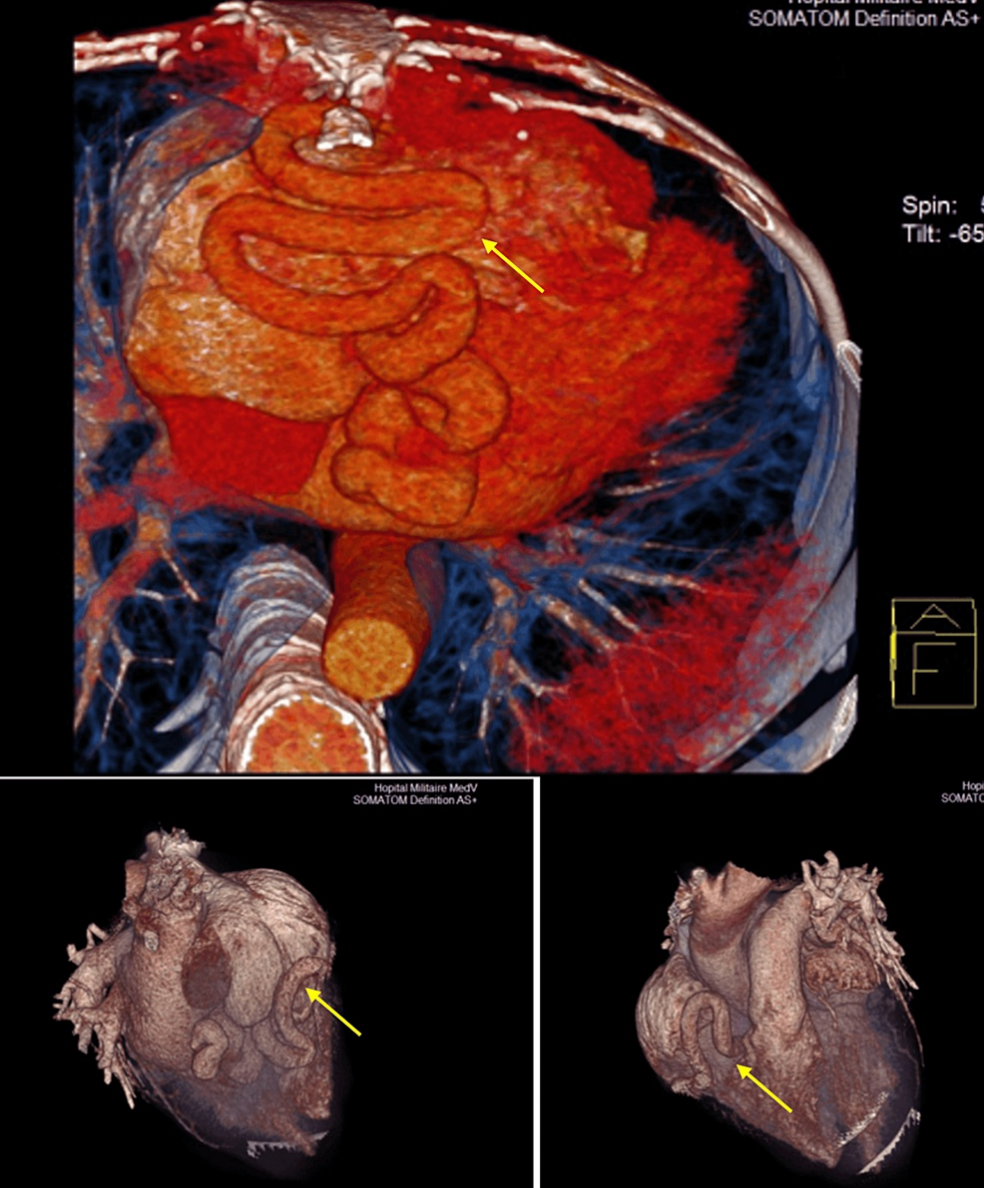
Voluminous and tortuous coronaro-cameral fistula developed between the RCA and right atrium diagnosed by multidetector CT using 3D volume-rendering images (yellow arrows)
